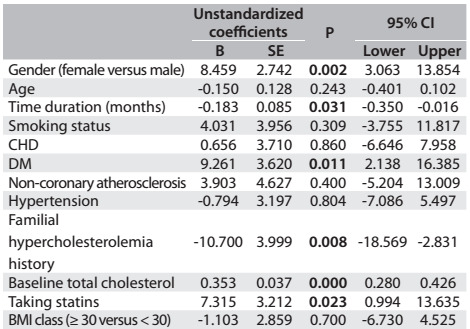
Magnetic resonance imaging of brain, three months after admission, showing: hyperintense image with T2 weighting (A); and enhancement after gadolinium-diethylenetriamine pentaacetic acid (Gd-DTPA) administration (B).
